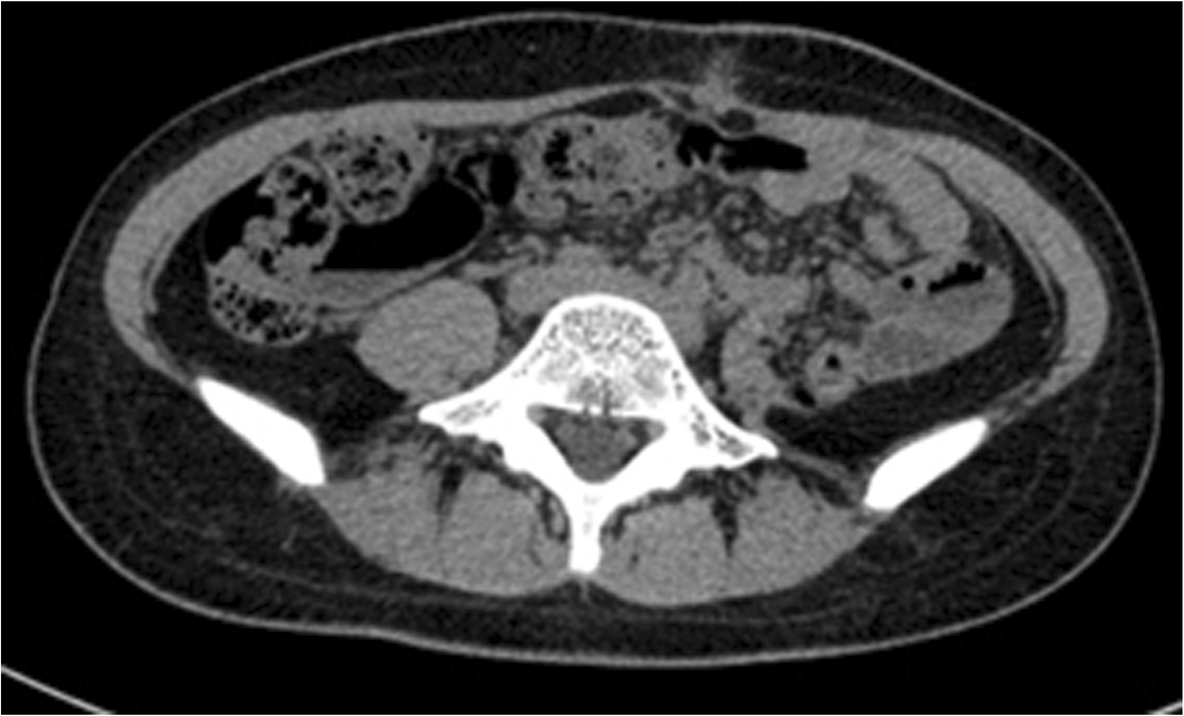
Follow-up CT 6 months following the surgery.
